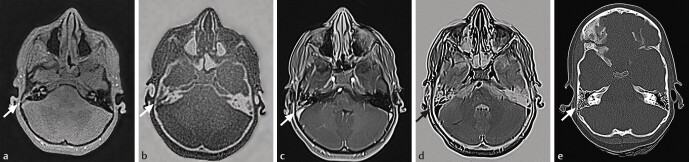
Comparison of ZTE vs. VIBE vs. CT for detection of mastoid fracture.
